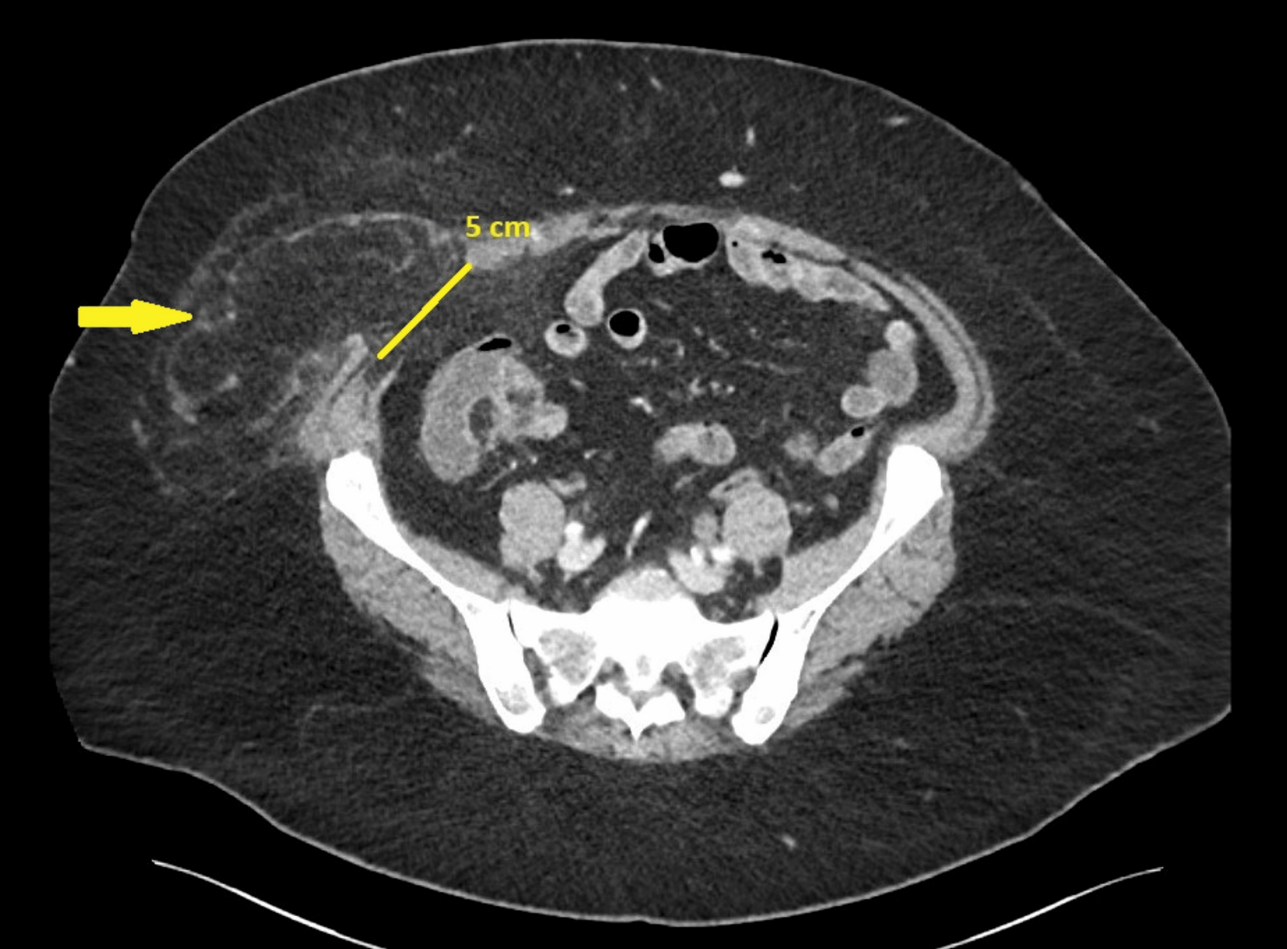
Axial CT scan demonstrating a right lower quadrant incisional hernia Axial CT image of the abdomen and pelvis showing a right lower quadrant incisional hernia (~5 cm) at the previous bowel extraction site from the 2019 laparoscopic sigmoid resection. The hernia sac contains omental fat without evidence of bowel incarceration. The yellow arrow indicates the location of the incisional hernia.
